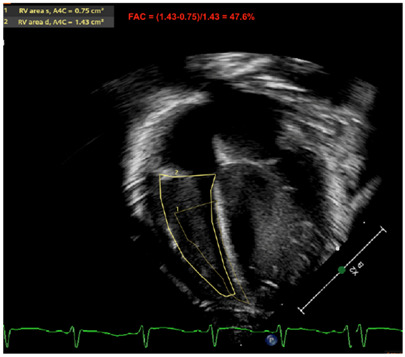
Legend: The fractional area change of the RV is another marker used to estimate RV systolic function. The contour at the endocardial border is traced at the peak of systole and at the peak of diastole. The fraction ([RV End Diastolic Area] − [RV End Systolic Area])/[RV End Diastolic Area] is expressed in % of area shortening. An apical RV focused view is used. This marker may be calculated in the apical four-chamber view. Some reports have also used the RV inflow–outflow view (or RV three-chamber view, or RV “tet” view—which outlines the RV anterior and inferior walls) to compute this metric, although the American Society of Echocardiography officially recommends its evaluation in apical four-chamber view—which outlines the RV free wall and RV septum. Here, the RV-FAC is 47.6%, which is considered normal (>35%)
